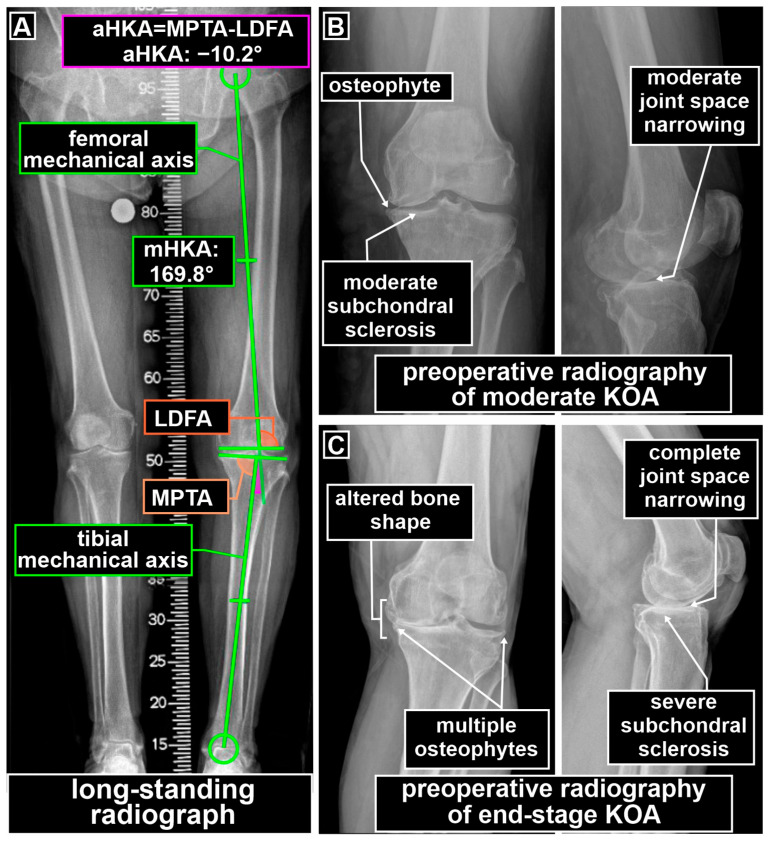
Representative preoperative radiographies of included individuals with primary KOA undergoing TKA. Readers should note that varus malalignment in the included individuals was confirmed by preoperative lower limb deformity analysis using long-standing radiographs (A). Patient with moderate KOA (Kellgren–Lawrence stage 3) preoperatively exhibits moderate osteophytes, joint space narrowing, and subchondral sclerosis (B), while a patient with end-stage KOA (Kellgren–Lawrence stage 4) preoperatively exhibits large osteophytes, severe to complete joint space narrowing, severe subchondral sclerosis, and a visibly altered shape of the femur and tibia (C). Abbreviations: KOA—knee osteoarthritis; TKA—total knee arthroplasty; mHKA—mechanical hip-knee-ankle-angle (angle between femoral and tibial mechanical axis); aHKA—arithmetic hip-knee-ankle-angle; LDFA—lateral distal femoral angle; MPTA—medial proximal tibial angle. [The figure is the author’s original work, arranged using vector graphic software (CorelDRAW Graphics Suite 21, Cascade Parent Limited, Ottawa, ON, Canada)].
